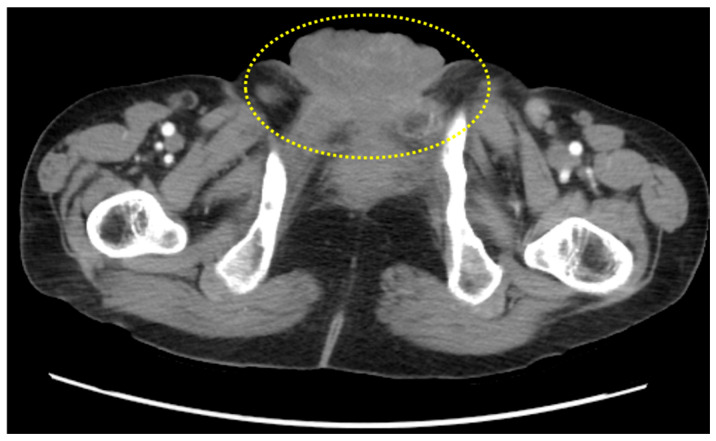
Computed tomography (CT) scan of the lower abdomen/pelvis demonstrating a midline tumor mass arising along the urachal tract.
